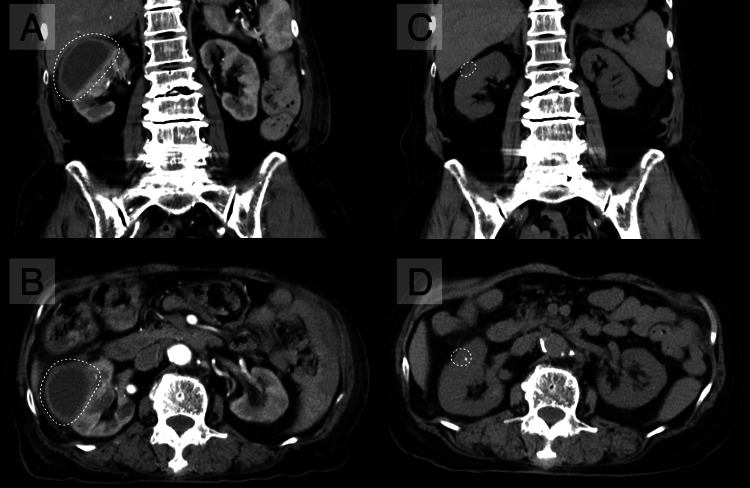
Follow-up imaging after treatment. A follow-up contrast-enhanced CT on day 34 showed a reduction in cyst size (67 × 50 × 33 mm, dotted circles), with no septa or enhancing soft-tissue components. (A) Coronal and (B) axial views. A follow-up non-contrast CT performed five months after discharge showed improvement of the enlarged cyst (dotted circles). (C) Coronal and (D) axial views.
